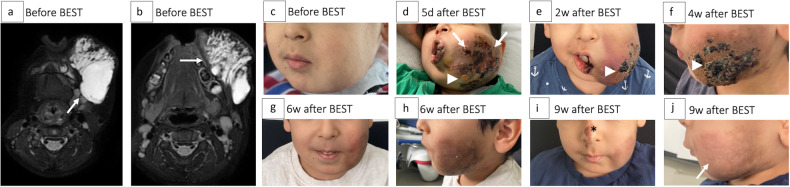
Four-year-old male patient with subcutaneous lymphatic malformation (LM) of the left cheek undergoing one bleomycin electrosclerotherapy (BEST). a, b T2-weighted, axial magnetic resonance images prior to treatment showing a partially micro- and partially macrocystic LM (arrows). c Clinical presentation of the patient prior to treatment including noticeable swelling of the affected region with consecutive mouth asymmetry. d Clinical presentation of the patient five days after BEST with extended postinterventional swelling (arrows) with consecutive dyspnea, which was strictly monitored, as well as skin alterations such as hyperpigmentation, necroses, and blebs (arrowhead). As oral food intake was not possible, the patient was fed intravenously, and prednisolone was administered. e–h The decreasing swelling and good healing of necrosis and blebs over time (arrowheads) while the distinct hyperpigmentation on the treated skin was pronounced on the maximum. i, j Significant reduction of skin hyperpigmentation six weeks after BEST (arrow) compared to (h)
